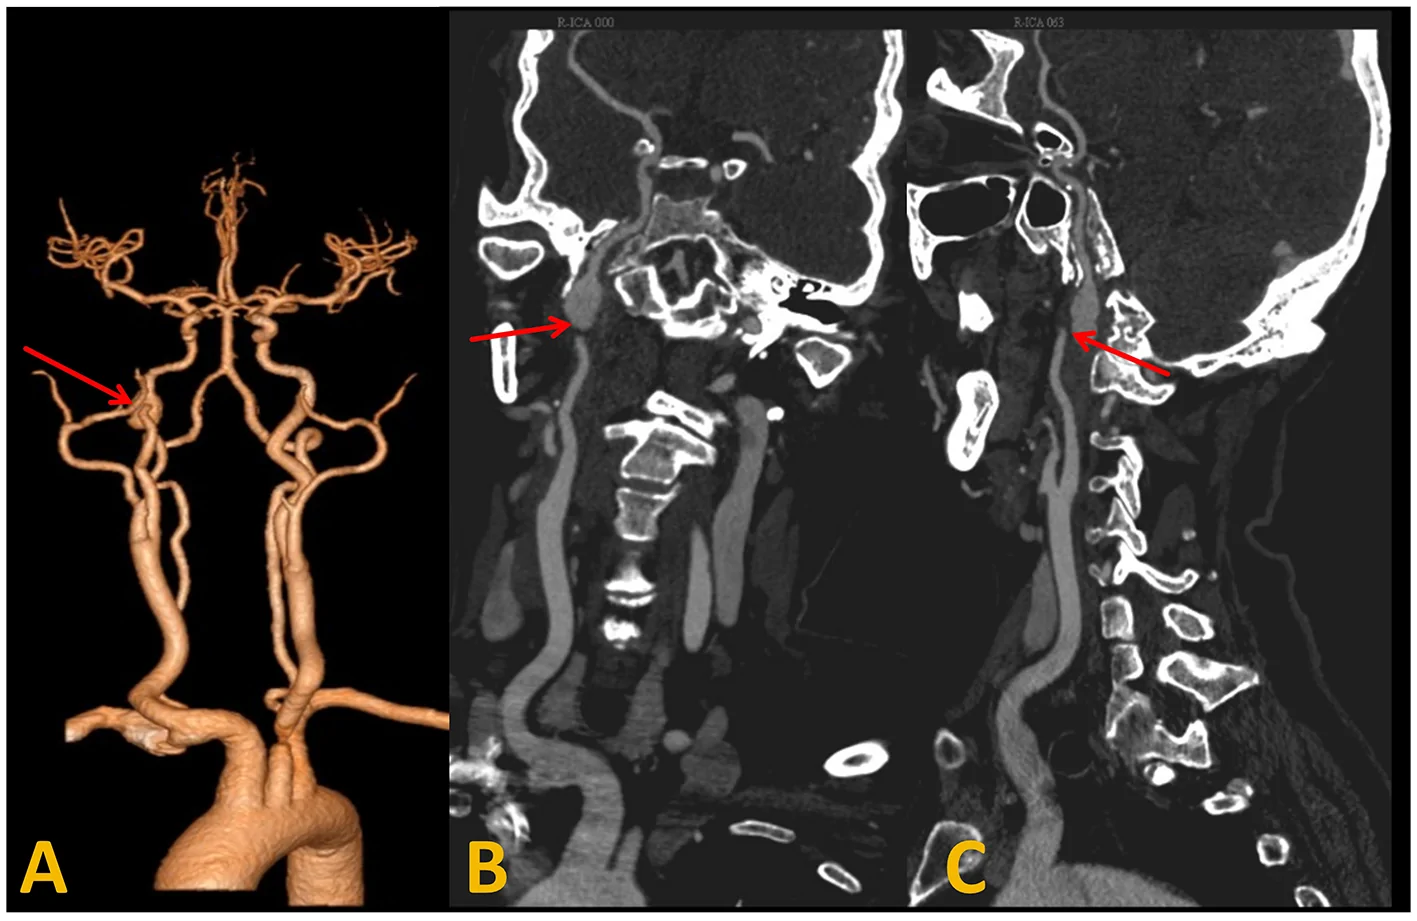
CTA of the head and neck on the day of admission. A, 3D-CTA; B, coronal 2D-CTA; and C, sagittal 2D-CTA of the head and neck taken on the second day of the patient's hospital stay. CTA indicates an aneurysm with severe proximal stenosis or near-total occlusion in the right internal carotid artery's C1–C2 segment.
